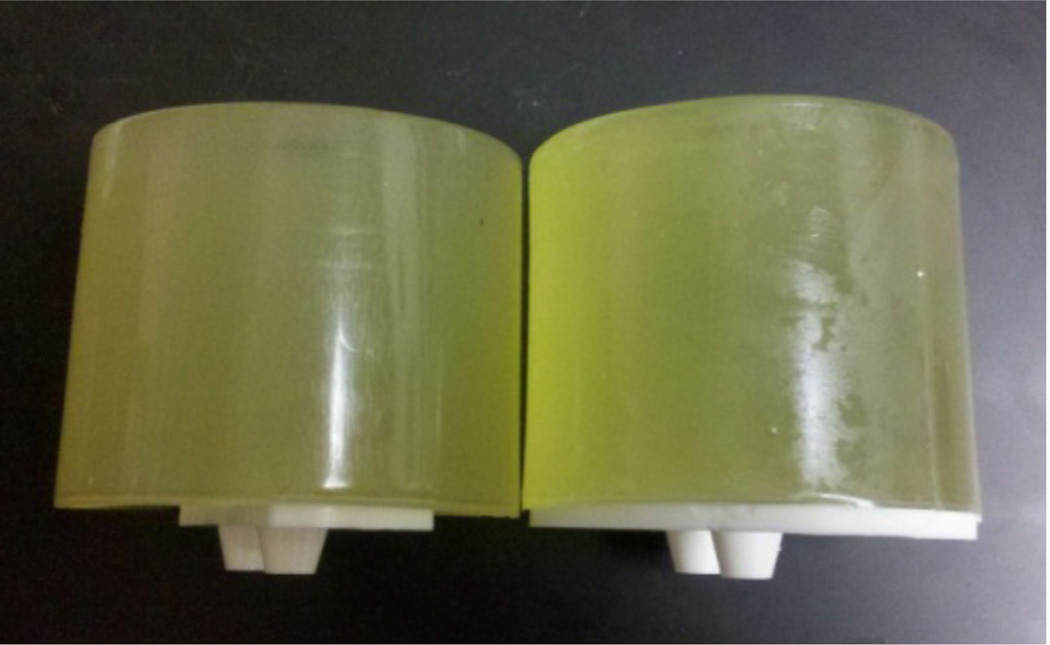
6 cm diameter cylindrical deformable dosimeters with attached plates keyed for directional registration in the optical-CT scanner.
